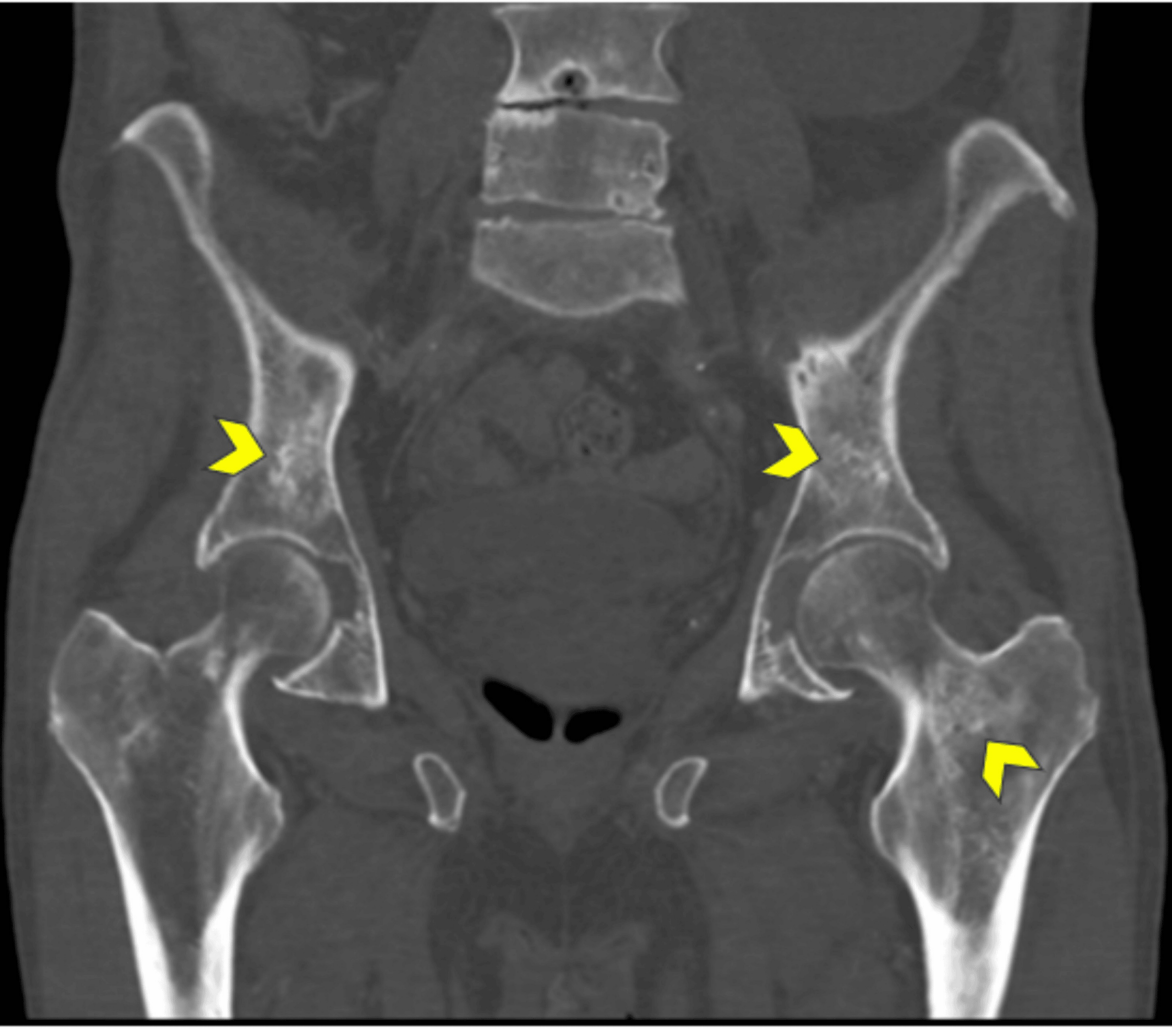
CT coronal reformation on bone window demonstrates small foci of osteosclerosis in both iliac wings as well in the femoral neck/proximal femoral metaphysis (yellow arrowheads). Degenerative changes of the lumbar spine are also seen CT: computed tomography
